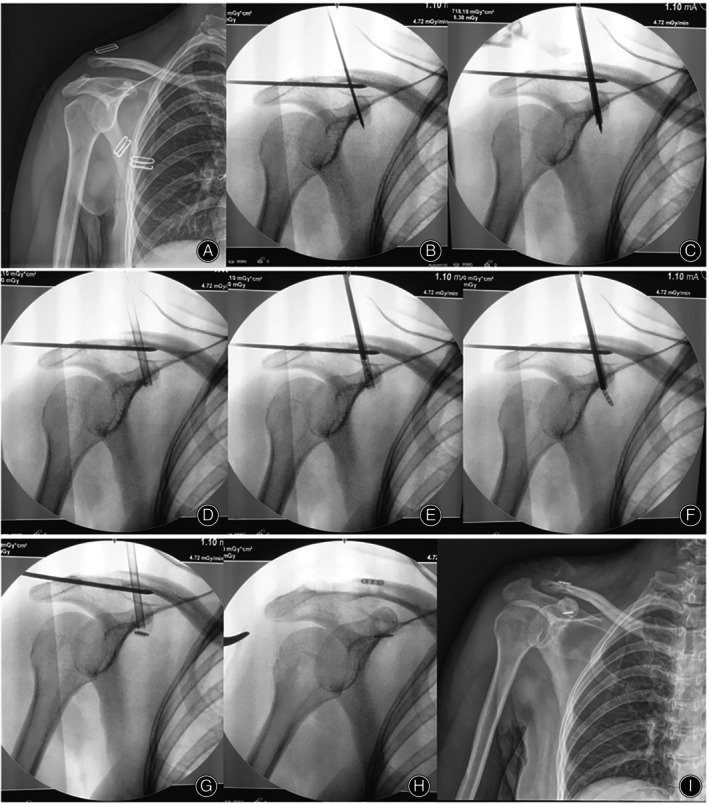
A 42‐year‐old woman with Rockwood type III ACJ dislocation. (A) Preoperative radiographs. (B) The ACJ is reduced and maintained by temporary K‐wires and a director is placed from the middle of the clavicle to the middle of the coracoid base. (C) A 4.6‐mm bony tunnel is drilled through the director. (D) The cannula is inserted through the bony tunnel from the clavicle to the coracoid. (E) A suture was placed through the Endobutton loop, and the Endobutton was then put into the cannula. (F) The Endobutton was placed at the coracoid base by the pushing rod. (G) The Endobutton gradually closed to the coracoid base by pulling the suture. (H) The second Endobutton plate without loops is placed in the loop above the clavicle. (I) Postoperative radiograph obtained to assess the reduction.
